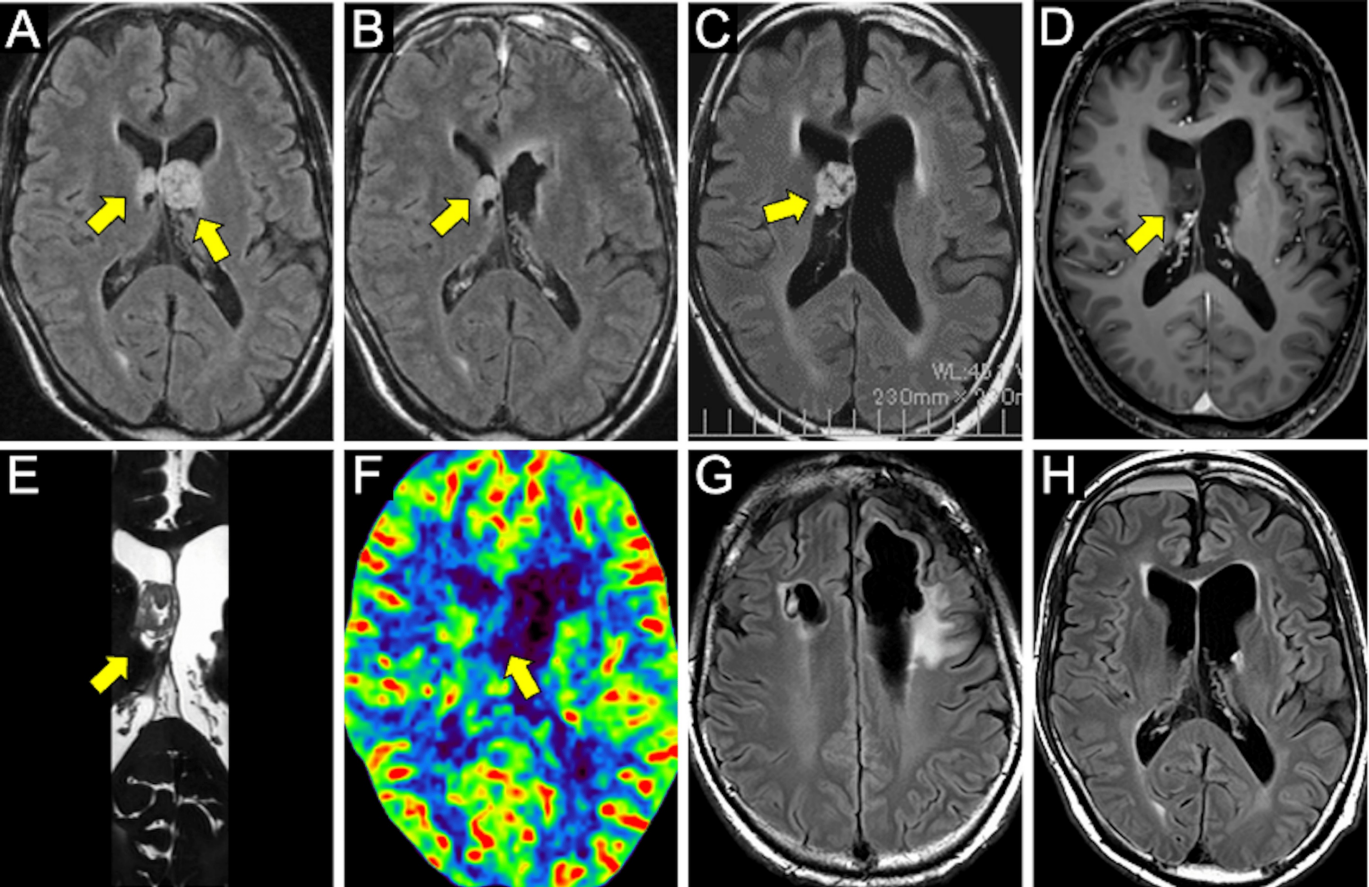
Images during the course of the disease A: FLAIR before initial surgery showing the tumor in the anterior horns of both lateral ventricles (arrow). B: FLAIR after the initial surgery showing the removal of the tumor on the left side and the persistence of the lesion on the right side (arrow). The images before the second surgery, FLAIR (C), Gadolinium contrast T1 (D), Heavy T2 (E), Perfusion CT (F) indicating that the tumor has grown compared to the previous examination (arrow); FLAIR 3 months after the second surgery showing uncapped left frontal lobe at initial surgery and narrow surgical corridor of right frontal lobe at the second surgery (G), no recurrence after gross total resection (H). FLAIR: fluid-attenuated inversion recovery
